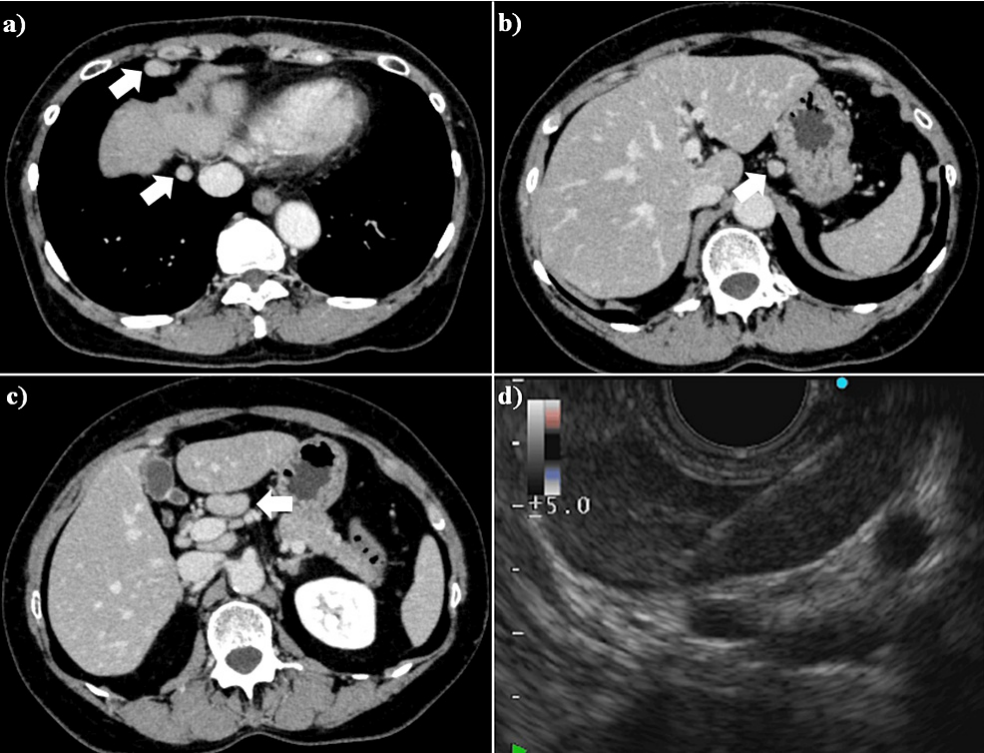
Computed tomography (CT) and endoscopic ultrasound (EUS) of the case 1 patient. Computed tomography (CT) shows the lymphadenopathy (arrows) affecting the a) supradiaphragmatic lymph nodes, b) gastric regional lymph node, and c) common hepatic arterial lymph node. d) Endoscopic ultrasound (EUS) shows lymphadenopathy as a hypoechoic mass lesion adjacent to the common hepatic artery. EUS-guided fine needle aspiration (EUS-FNA) of the lymphadenopathy is performed.
